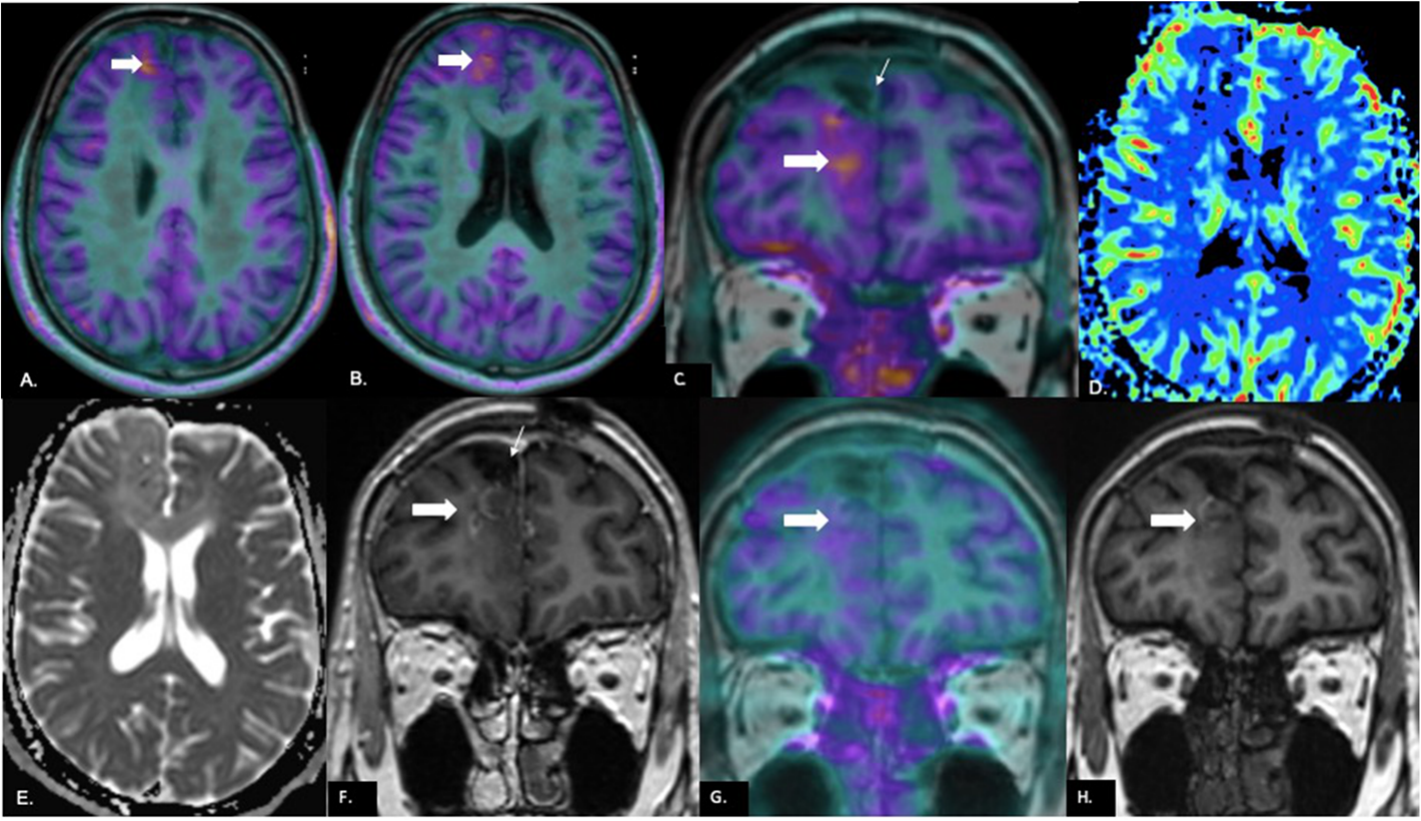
Discordance between PET, perfusion and diffusion in a case of recurrence: 31-year-old male operated for right frontal ODG 14 months back; last radiation dose 10 months ago. PET images fused with axial T1 MPRAGE (A, B) show an area of uptake in the right superior frontal gyrus (white arrow in A, B). The uptake (block white arrow) is seen along the margins of the resection cavity (small white arrow) on the coronal fused PET-MR image (C). rCBV map (D) generated using the leakage correction algorithm shows no unequivocal elevation of perfusion in the area. There is no diffusion restriction on the ADC map (E). Few foci of enhancement (block white arrow) are seen along the margins of the resection cavity (small white arrow) on post-contrast coronal T1 MPRAGE (F). In view of PET uptake, recurrence was suspected and chemotherapy administered. Follow-up PET image after chemotherapy fused with coronal T1 MPRAGE (G) shows reduction in the degree of uptake (block white arrow) along the resection cavity margin when compared with prechemo coronal PET image in C. Reduction in the degree of enhancement is also seen on the follow-up post-contrast coronal T1 MPRAGE (white arrow in H) compared to that in F
